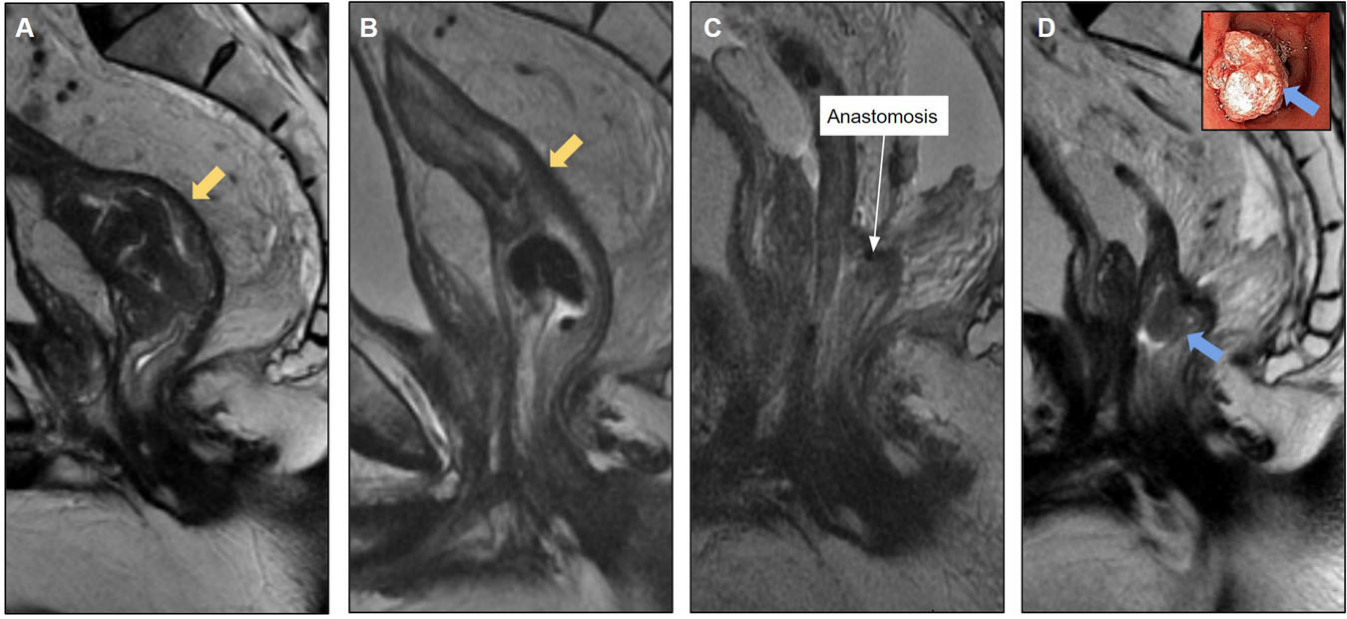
Case of anastomotic recurrence. A Sagittal T2-weighted image: baseline mrT2N1 (yellow arrow), mesorectal fascia (MRF) clear and no extramural vascular invasion (EMVI). B Post-long-course chemoradiotherapy (CRT) restaging shows response tumor regression grade (TRG) 2 (yellow arrow). C Follow-up after mesorectal excision (ypT2N0), with clear circumferential resection margin (5 mm) and insufficient distal margin: 1.6 cm. D Four months after surgery. Colorectal anastomosis recurrence was detected by endoscopic examination (blue arrow). MRI to plan surgical resection. Absence of sacral, neural, lateral wall and other adjacent organs invasion. Rectosigmoidectomy for anastomotic recurrence resection was proposed
